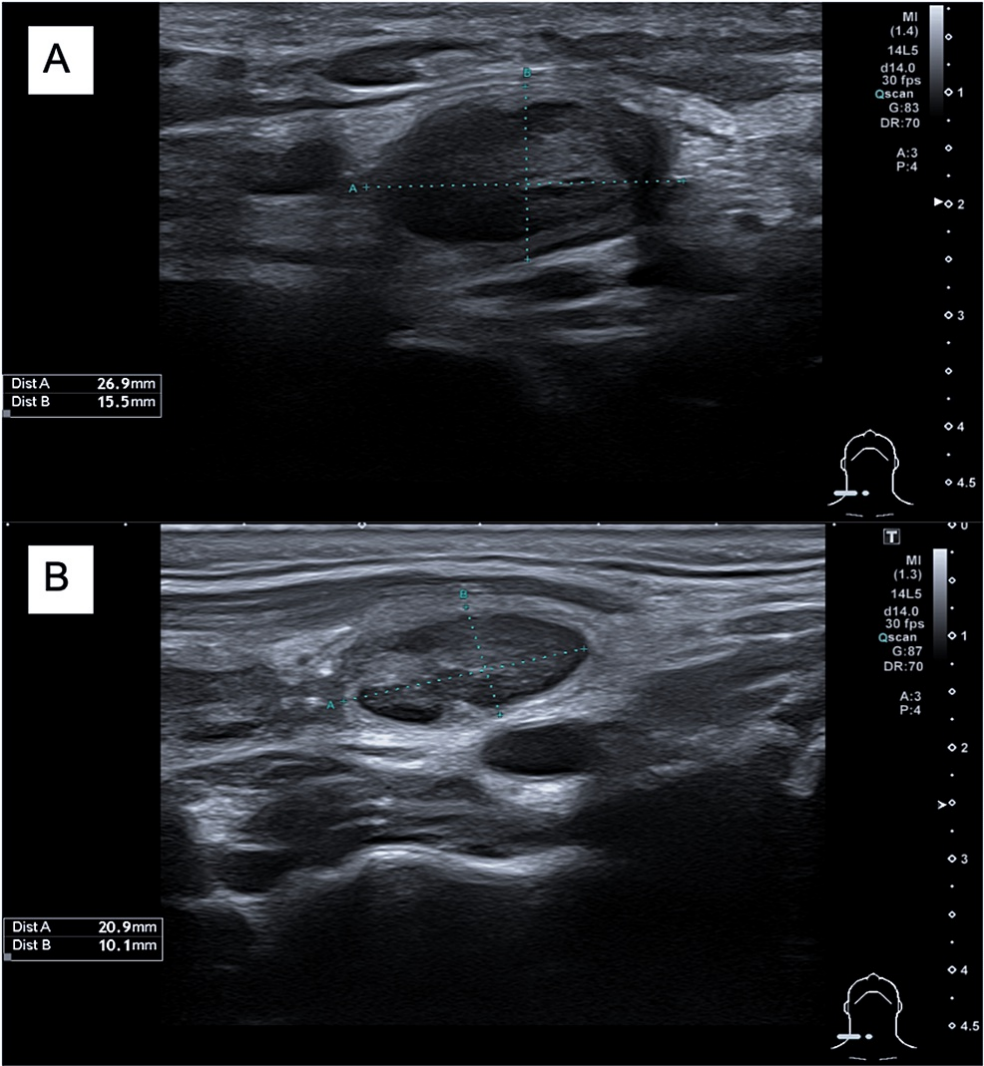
Neck ultrasound Adenopathy in the right lateral cervical region with globose morphology and hypertrophy of the cortical area: the largest ones with 27 x 16 millimeters (A) and 21 x 10 millimeters (B).
